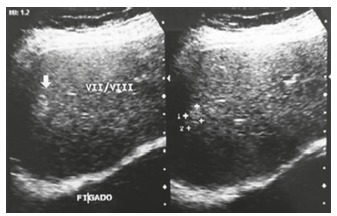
Ultrasonography with nodular image suggestive of hemangioma in segment VII/VIII
